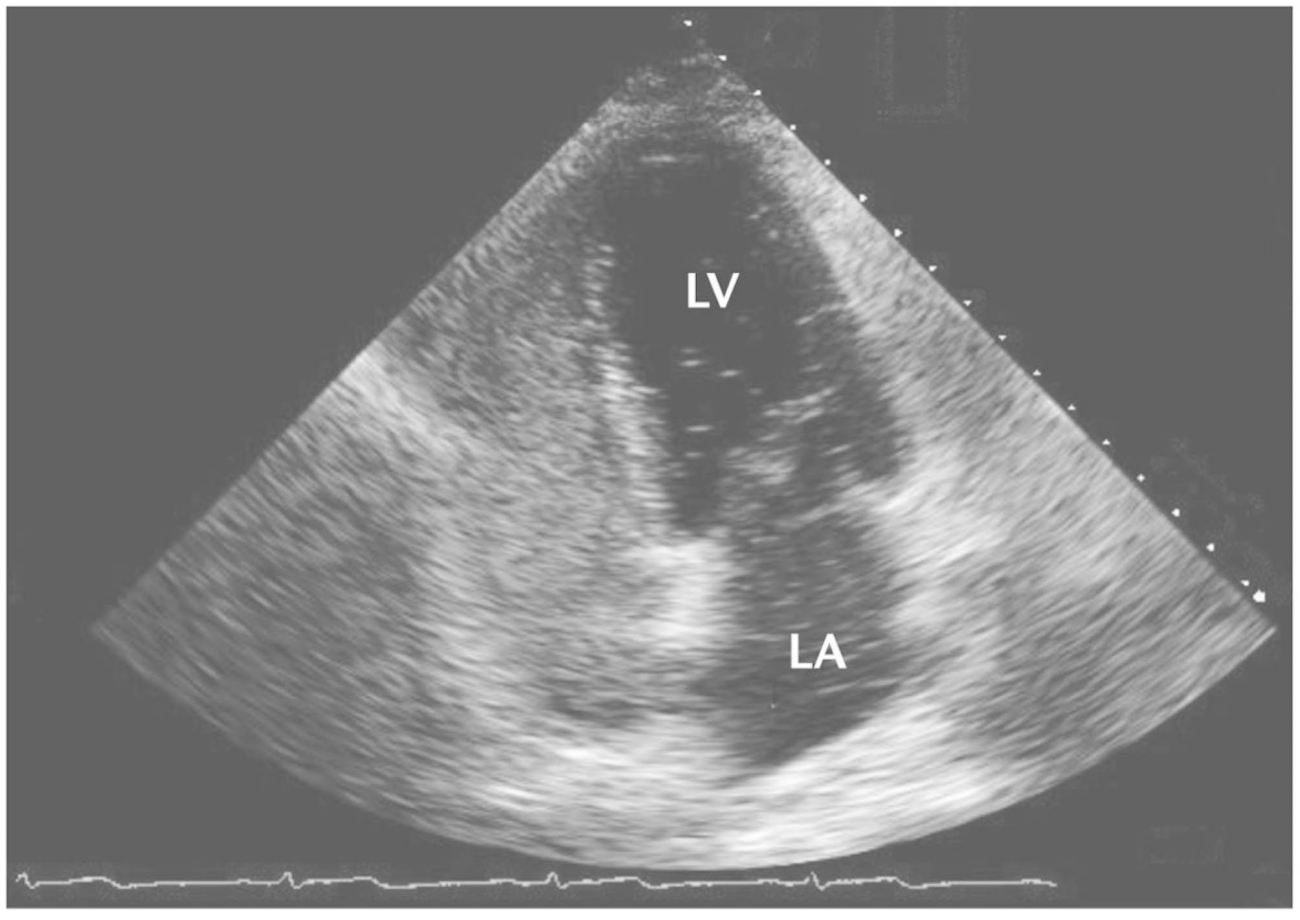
PFO detection by transthoracic echocardiography with contrast injection. Note: The right-sided cardiac chambers are completely opacified by contrast material (aerated saline) and shunting microbubbles are visualized in the left atrium (LA) and the left ventricle (LV). PFO, patent foramen ovale
